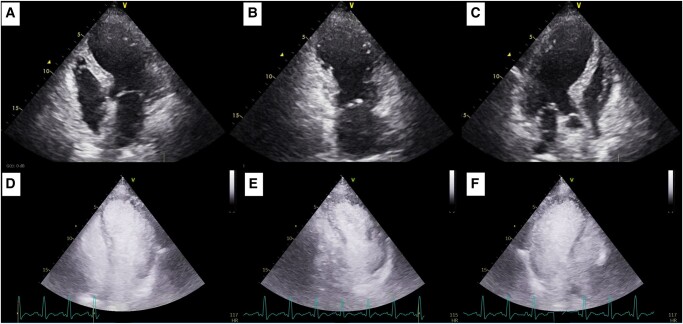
Transthoracic echocardiogram at admission exhibiting a non-dilated left ventricle with severely reduced ejection fraction (25%). (*A*) Apical four-chamber view. (*B*) Apical two-chamber view. (*C*) Apical three-chamber view. Transthoracic echocardiogram with SonoVue® contrast excluding left ventricular thrombus and rupture. (*D*) Apical four-chamber view. (*E*) Apical two-chamber view. (*F*) Apical three-chamber view.
